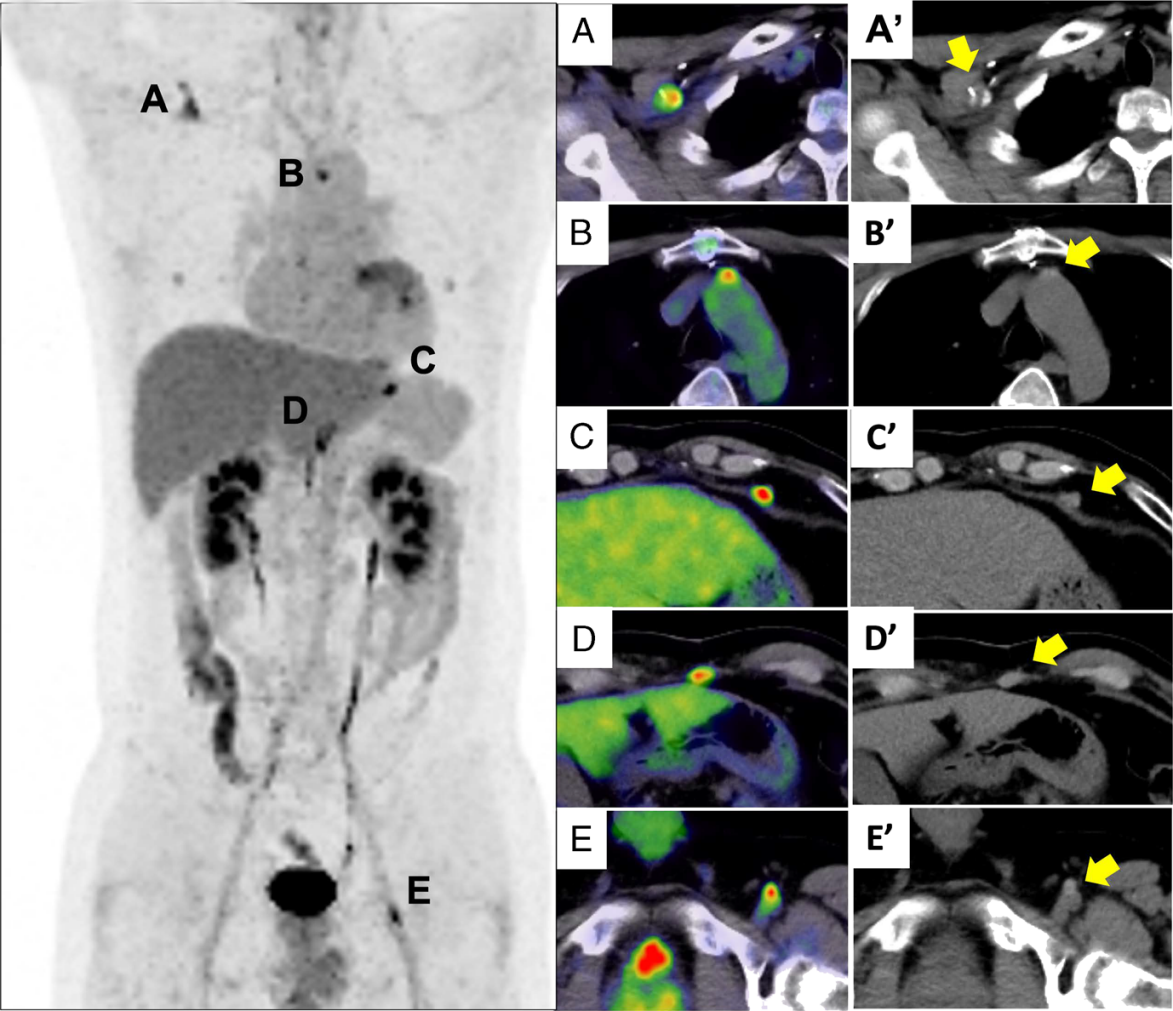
A 37-year-old man with a history of heart transplantation underwent ^18^F-FDG PET/CT in screening for malignancy under posttransplantation immunosuppression. He had no clinical symptoms and no blood data abnormalities. The screening images demonstrate FDG uptake in 2 soft tissue density nodules in the peritoneum (C, D, C′, and D′).
